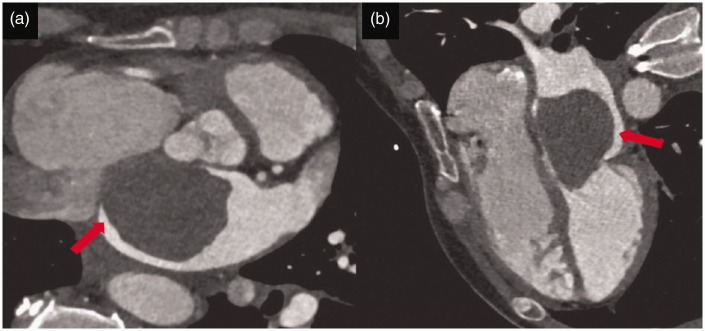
(a) Axial and (b) sagittal cardiac computed tomography images showing a large left atrial myxoma (red arrows) with diastolic prolapse into the left ventricle (used with permission).
